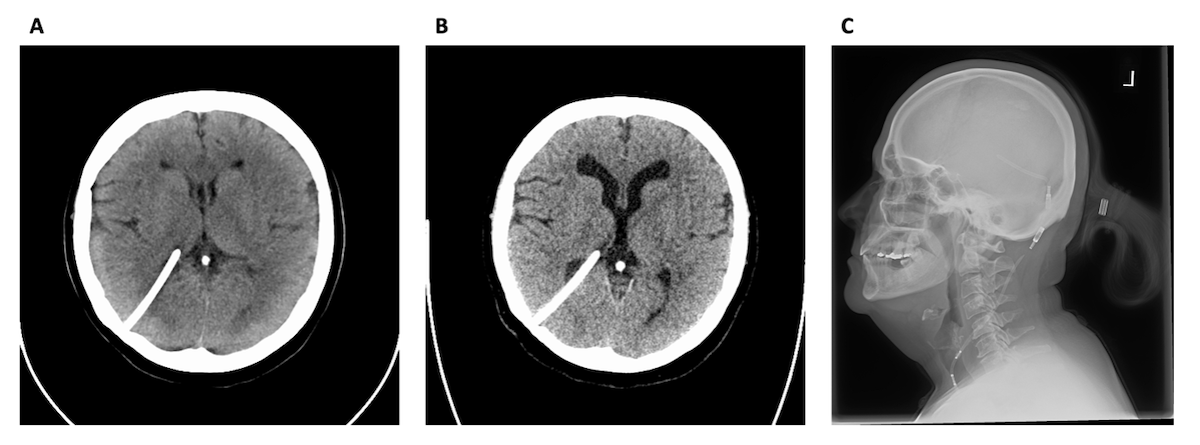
Investigations supporting hydrocephalus and shunt dysfunction: (A) CT scan from 13 years before the revision; (B) CT scan 1 month before the revision; and (C) shunt series x-ray 1 month before the revision. CT: computed tomography.
